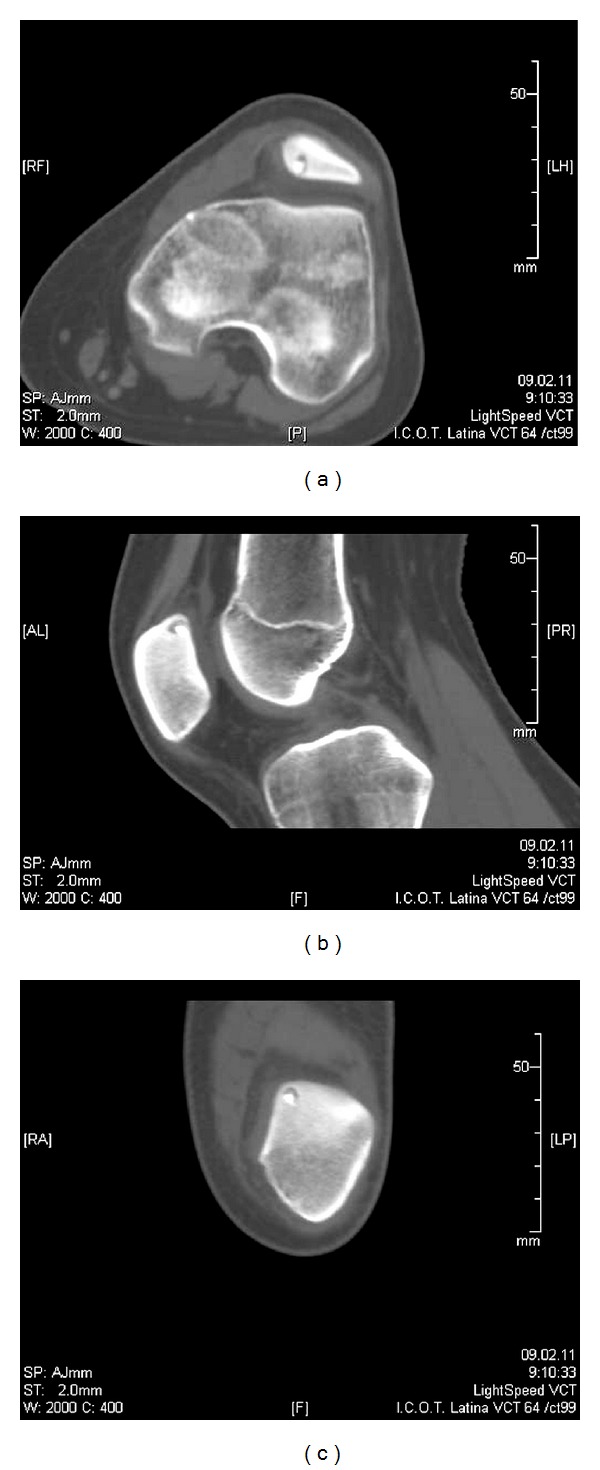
(a) Axial, (b) sagittal, and (c) coronal CT scans show a round, well-marginated sclerotic lesion of about 8 mm with a hypodense rim and a centrally calcified nidus.
